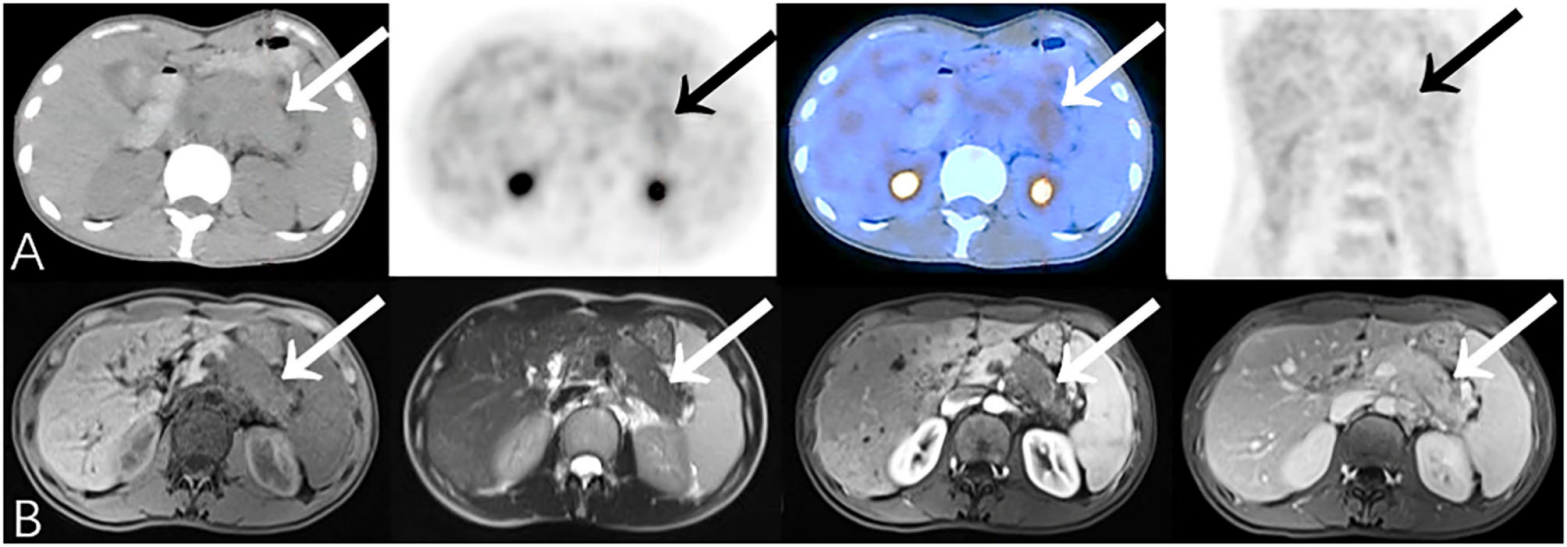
Representative patient on PET/CT (False-Negative) compared to CEMR (True-Positive): A 37-year-old female experienced epigastric pain for 4 months. Serum CA19-9 was elevated (259.4 U/mL). (A) The body and tail of pancreas were slightly swollen with slightly increased ^18^F-FDG uptake (SUVmax 3.1). There was insufficient evidence of malignancy on PET/CT especially when no other significant abnormality was identified on the rest of the body scan. (B) CEMR showed a low-signal area on T1WI with narrowing of the pancreatic duct in the body and tail of pancreas which has no significant density change on PET/CT due to relatively limited resolution. The lesion enclosed retroperitoneal vessels which was suggestive of pancreatic cancer. Biopsy result showed adenocarcinoma (differentiation unspecified). Patient died after 9.2 months.
